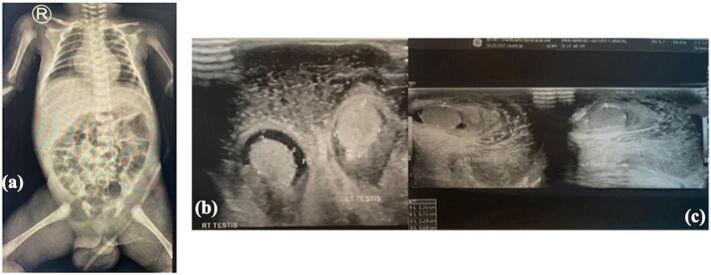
Abdominal X-ray showed a gas appearance in the scrotal region.
